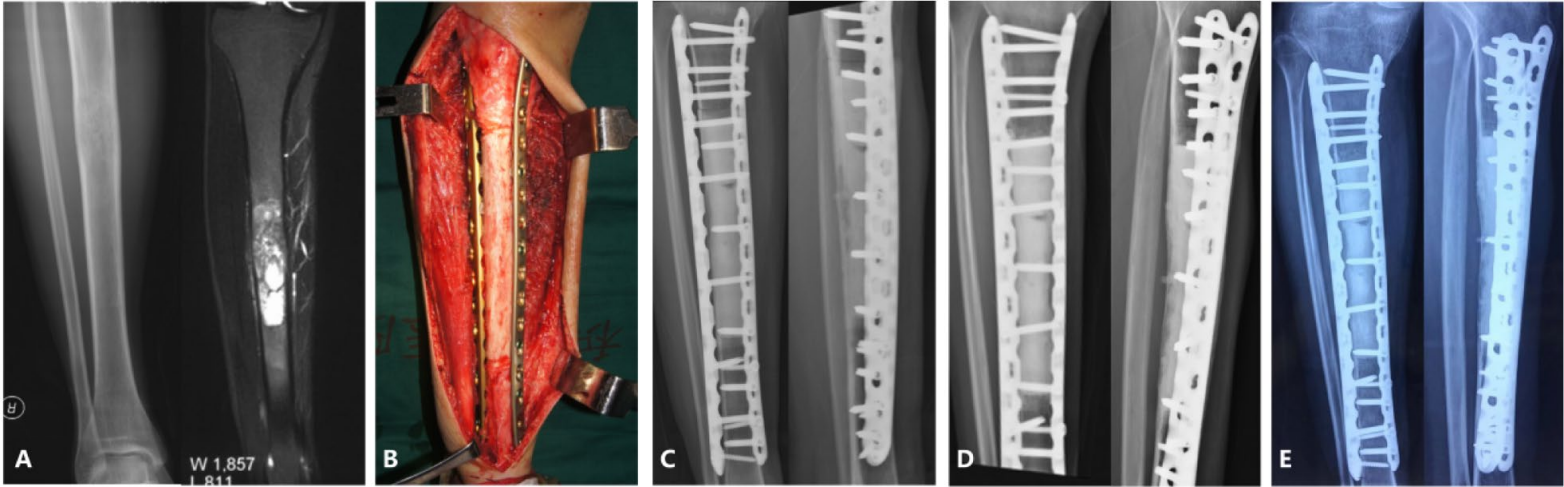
A 30-year-old male with Ewing sarcoma of the right tibia. A X-ray and T2-weighted MRI showed tumor was located in the right tibial diaphysis. B The autogenous bone was inactivated in liquid nitrogen and reconstructed with bilateral bridging plates. C Immediate postoperative image shows bridging plate fixation combined with intramedullary cement. D At 10 months postoperatively, the proximal and distal osteotomy sites had excellent consolidation. E Five years after surgery, the autograft is still in good condition
